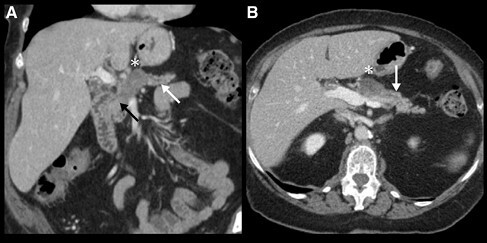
Figure 1. Contrast enhanced CT demonstrating typical finding in DPDS in coronal (A) and axial (B) views. CT demonstrates 3cm area of necrosis involving pancreatic neck/body (asterisk) with viable upstream parenchyma in the remainder of the body and tail (white arrow). Pancreatic head/uncinate parenchyma and main duct appear intact (black arrow). B, the distal pancreatic duct is not dilated. *DPDS, disconnected pancreatic duct syndrome.*
